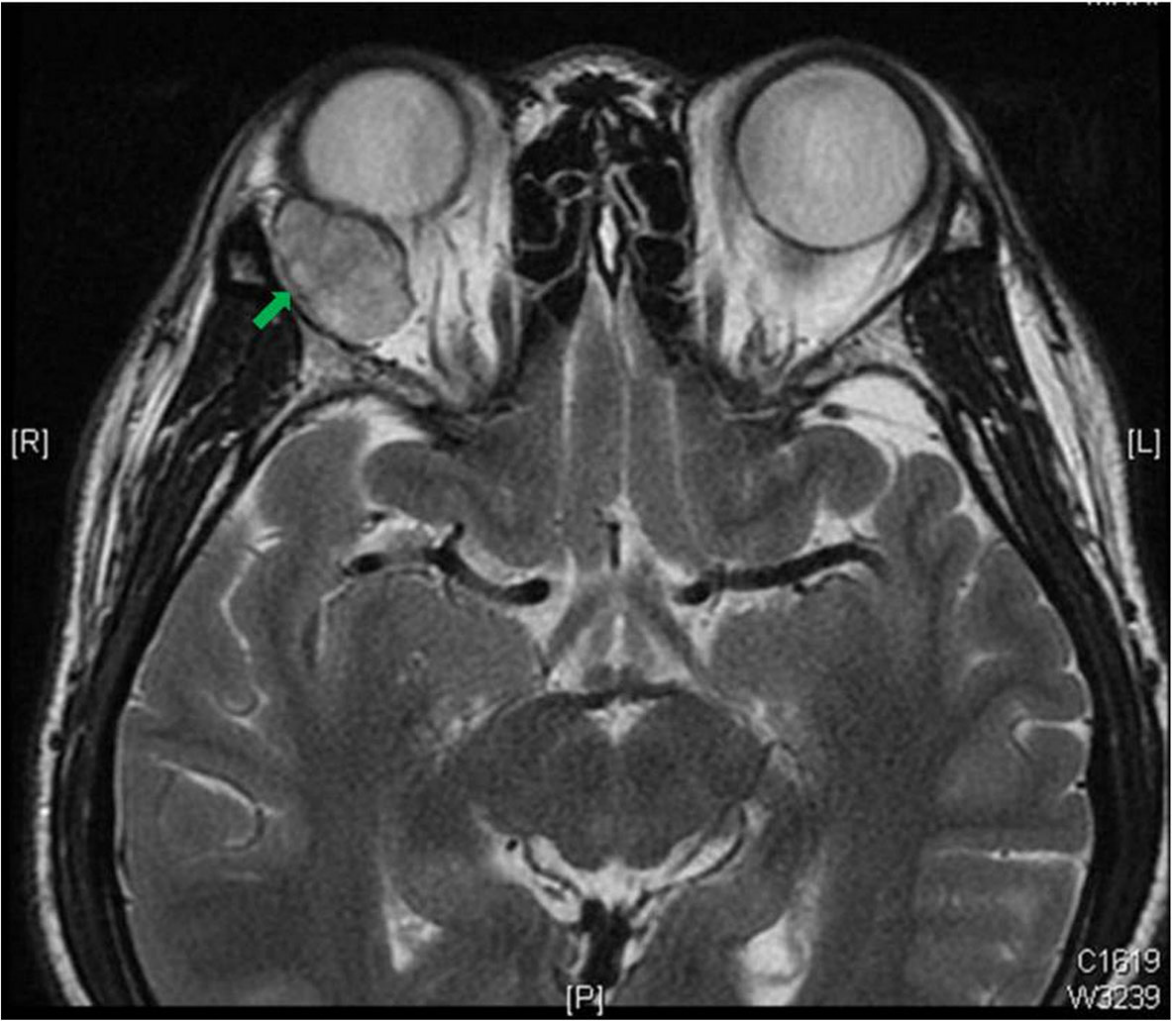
Magnetic resonance imaging (MRI) image of the patient. Magnetic resonance imaging (MRI), T2WI, axial view showed a well-defined, oval shape right intraconal tumor that hyperintensity signal with contrast enhancement about 16 × 24 × 22 mm in size (arrow)
